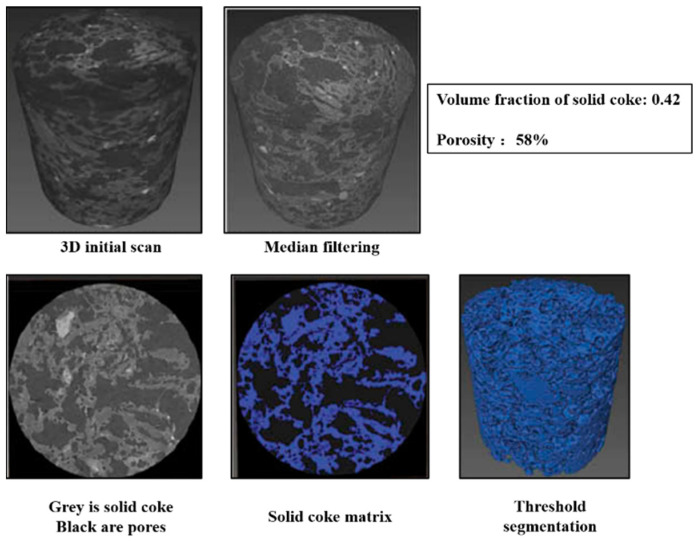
The pore distribution of coke samples in X-ray imaging [80].
